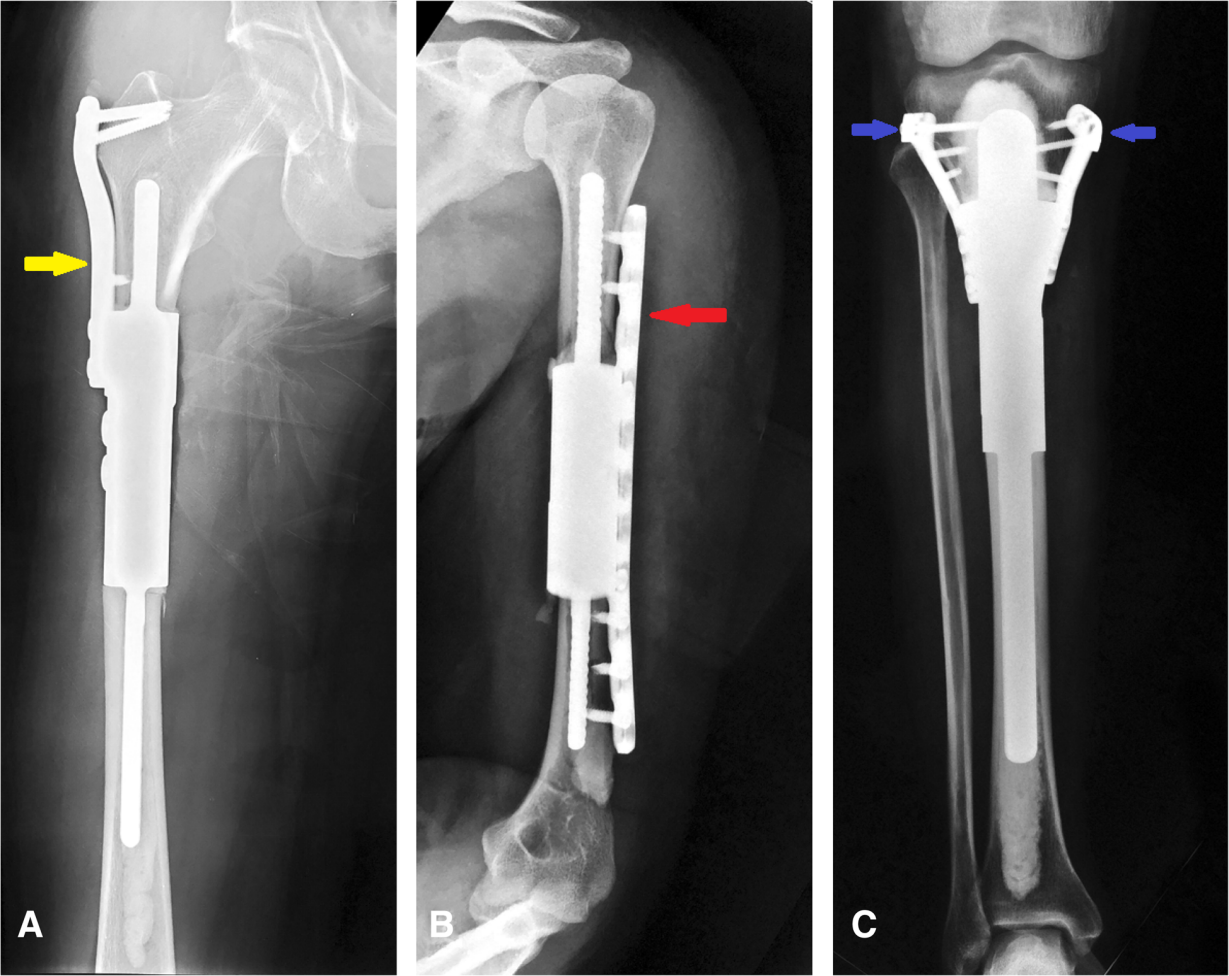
All prosthesis intramedullary stems were fixed with bone cement and an assistant plate was used when the intramedullary stem was shorter than 5 cm. Radiographs evidencing the use of assistant plates in (a) the femur and (c) tibia. The yellow arrow in a showing the unilateral assistant plate used in the proximal femur. The biomechanical analysis showed that the use of assistant plate improved the rigidity of anti-tension and anti-torsion, and diminished the risk of prosthetic loosening and dislocation. The use of an assistant plate in the humerus can effectively reduce the stress and the risk of loosening of the prosthesis. The red arrow in b showing a long assistant plate used in humerus. In this study, 12 patients accepted assistant plate fixation of the prothesis, including 5 for the humerus, 6 for the femur, and one for the tibia
